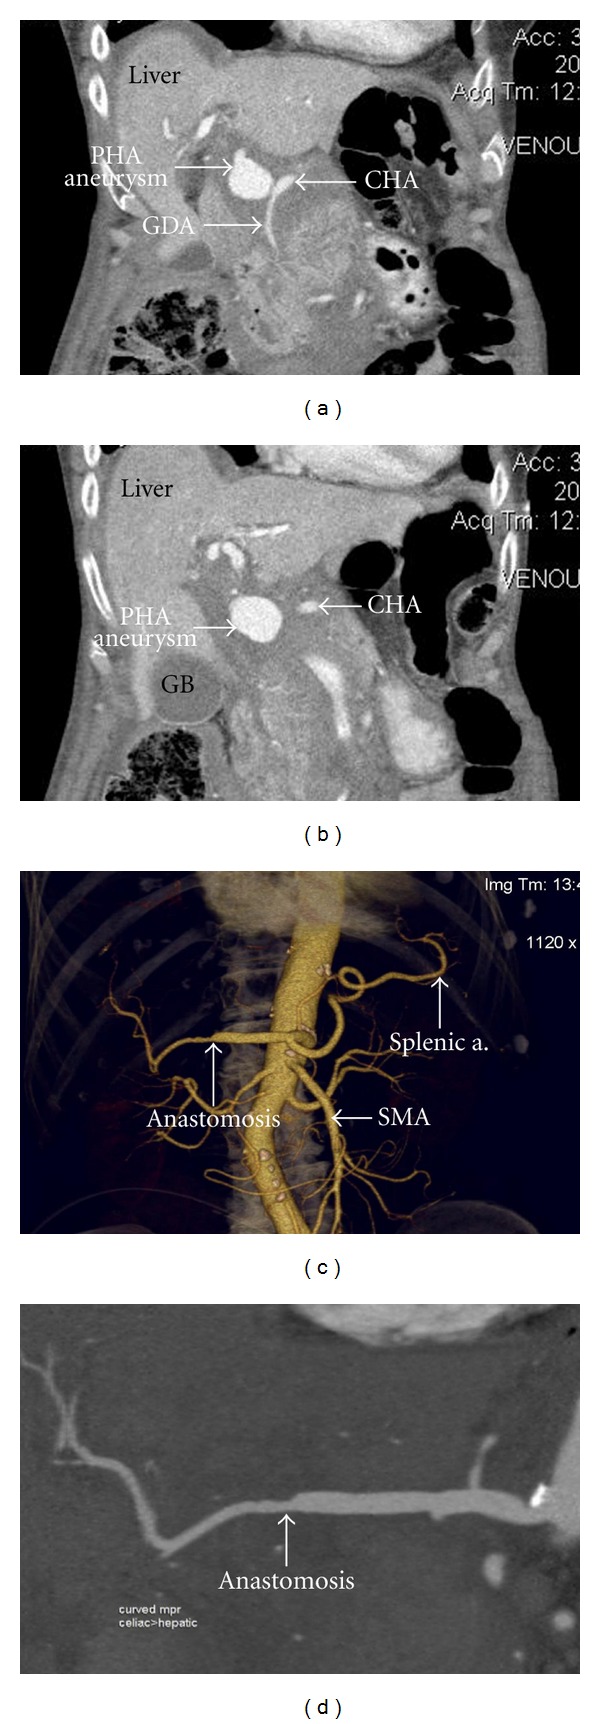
((a), (b)) Coronal CT scan depicting PHA aneurysm extending from the origin of the GDA to the bifurcation of the RHA and LHA. (c) Three dimensional CT reconstruction showing anastomosis between remaining PHA and RHA. (d) Enlarged view of coronal CT scan after anastomosis was completed.
